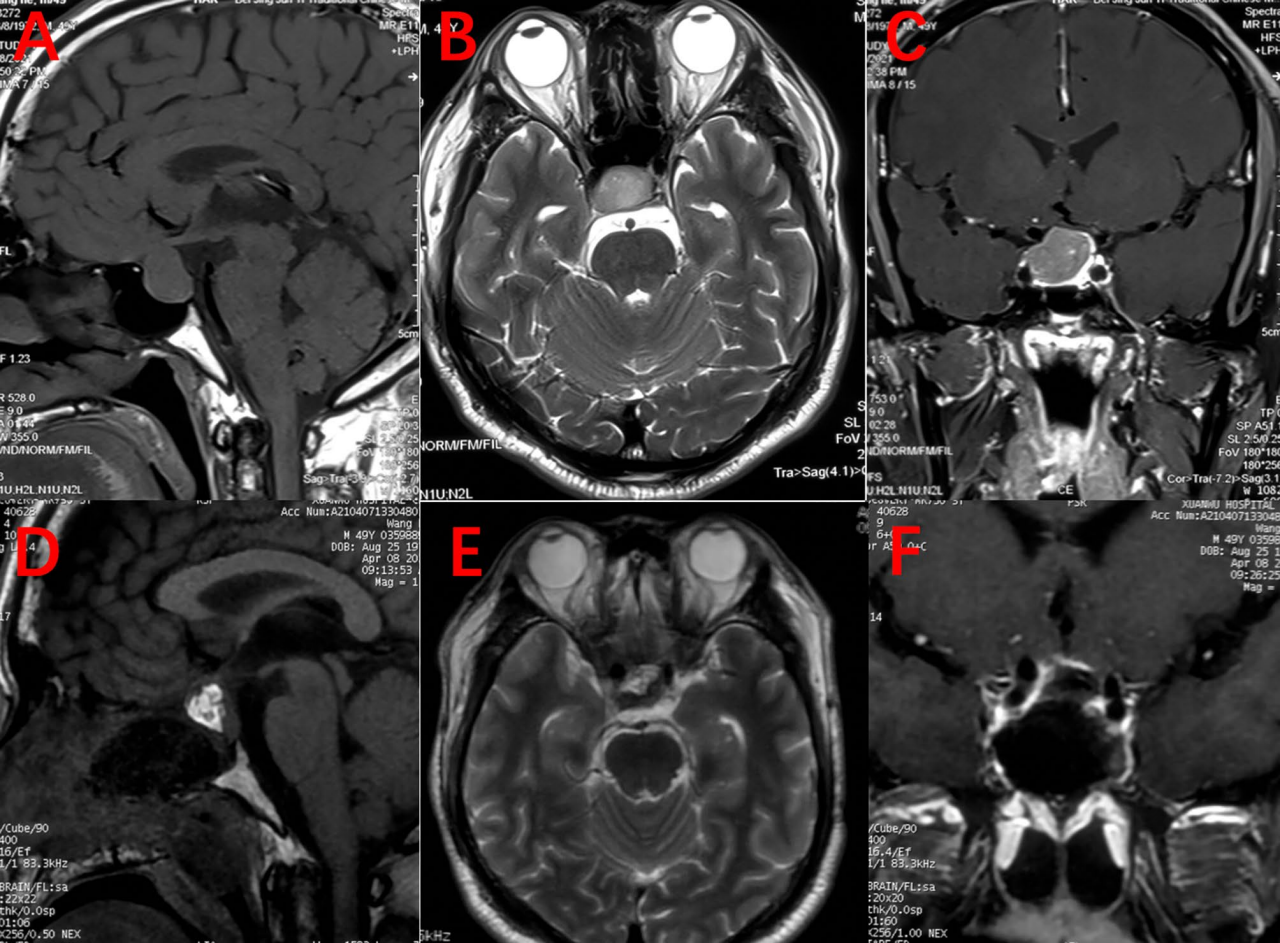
A-C was preoperative cranial MRI, the sellar tumor was visible, the optic chiasm was pushed upward by the tumor, and the normal pituitary gland was pushed to the left side. D-F was postoperative MRI, which observed that the tumor was completely excised, the autologous adipose tissue was packed in the sella turcica, and the normal pituitary gland was preserved
